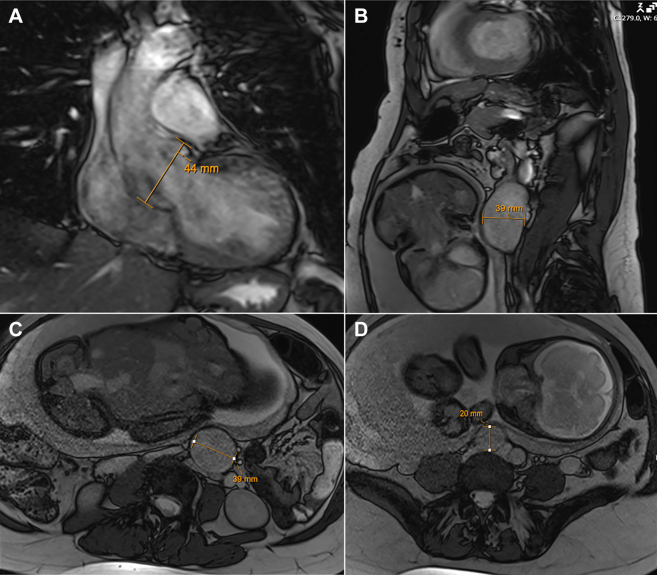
Preoperative MRI of the Chest, Abdomen, and Pelvis Preoperative MRI demonstrating (A) 4.4-cm aortic root, (B and C) 3.9-cm infrarenal abdominal aortic aneurysm, and (D) 2.0-cm right common iliac artery aneurysm, characteristic of the diffuse aneurysmal disease seen in Loeys-Dietz syndrome. MRI = magnetic resonance imaging.
